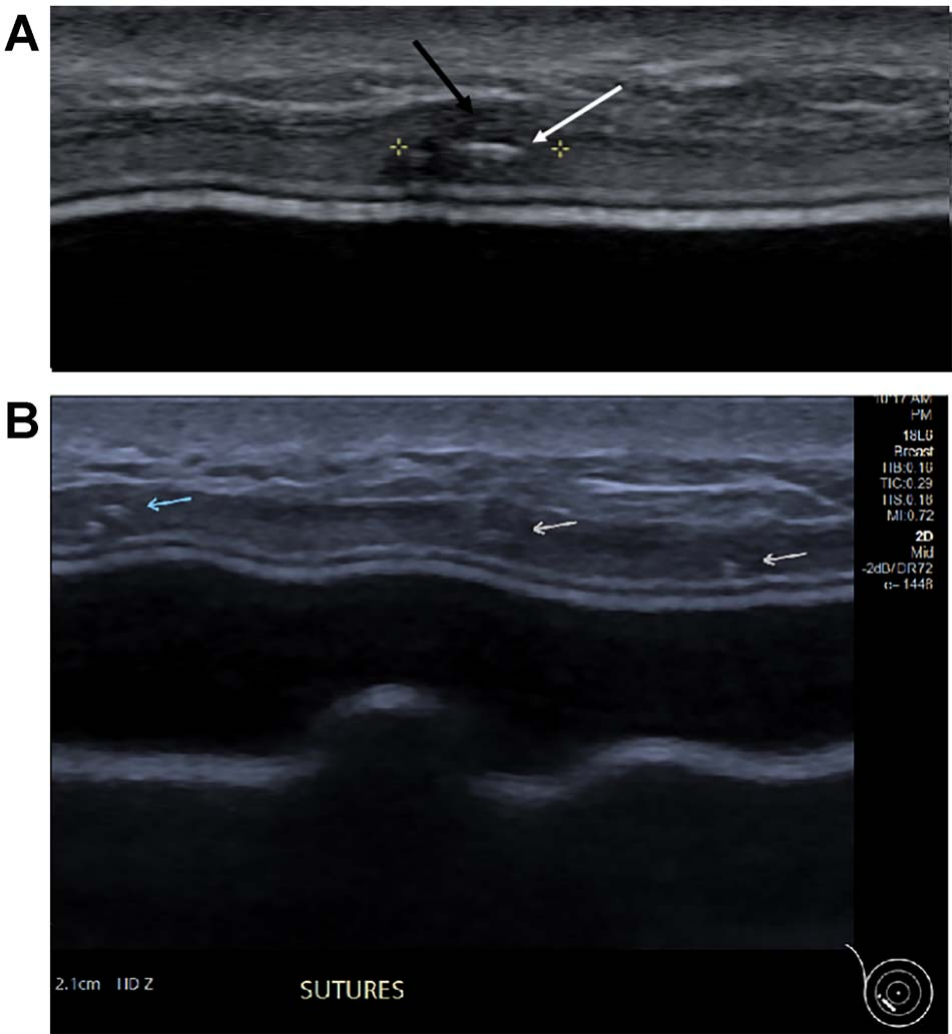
(A) Magnified ultrasound image identified a 5 mm hypoechoic focus of thickening within the capsule (black arrow and area between the cursers) with two parallel lines centrally within the focus which represents the suture (white arrow). (B). The ultrasound demonstrates the equally placed sutures identified as small white parallel lines (arrows) across the ADM.
